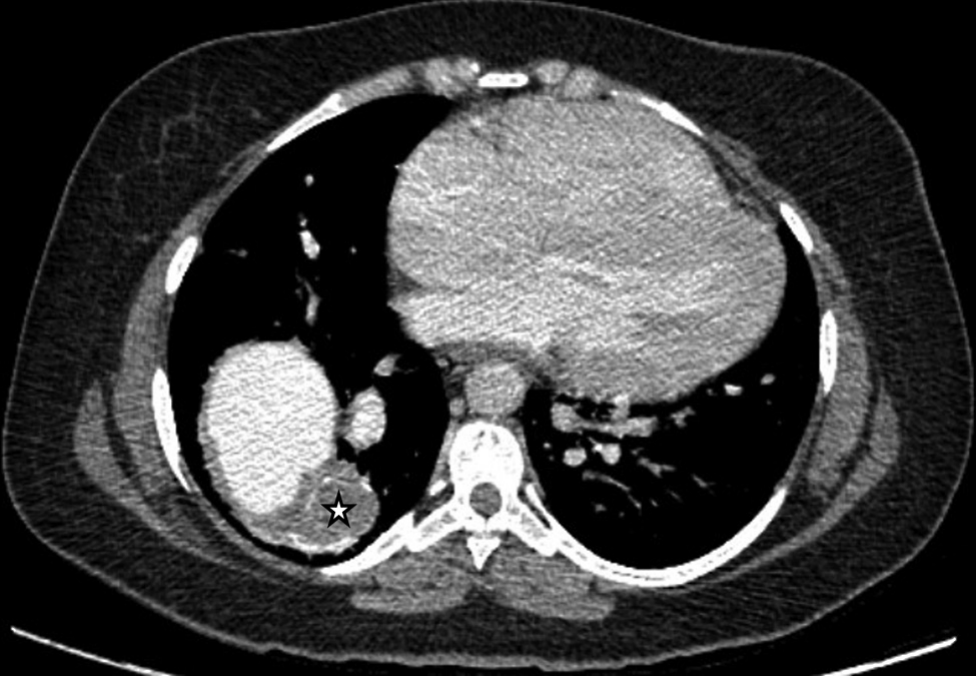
Delayed phase axial computed tomography angiography (CTA) shows a partially thrombosed right lower lobe pulmonary artery with peripheral calcification (star). This corresponds to the mass noted on the chest radiograph.
